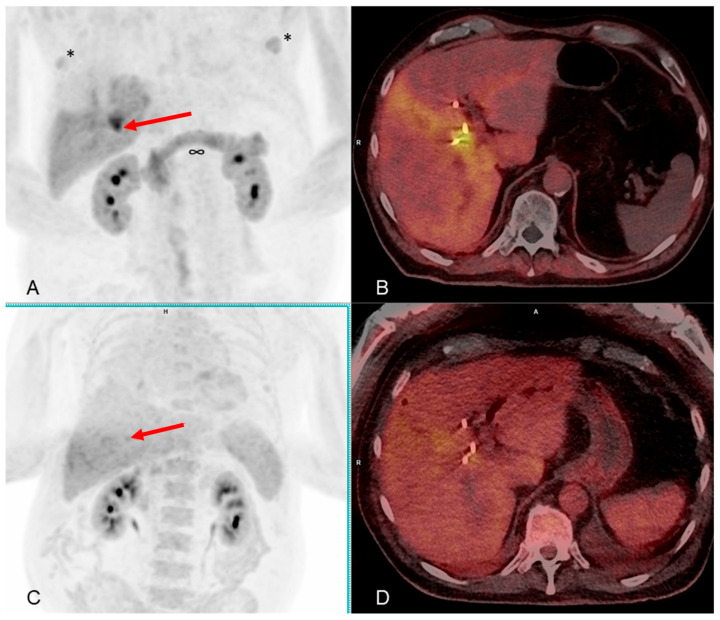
(A,B) MIP and hybrid PET/CT scan slice from December 2023 using [^68^Ga]Ga-FAP-2286: the red arrow marks an intense focal tracer uptake, which has been interpreted as the primary tumor lesion atop the confluence of the right and left hepatic bile ducts (Klatskin tumor). SUV_max_ 13.7; SUV_peak_ 10.7. (C,D) MIP and hybrid PET/CT scan slice from November 2023 using [^18^F]FDG: the red arrow marks a slight, inhomogeneous background noise enhancement at the confluence of the right and left hepatic bile ducts. However, the entire liver tissue shows this inhomogeneous uptake pattern. There is no clear focally-increased, neoplastic uptake delineated. Please take note of the liver parenchyma’s unevenly dispersed FAP expression, which has been interpreted as reactive uptake after acute cholangitis. It is important to take attention of the diffuse expression of FAP in the pancreas (∞), which has been attributed to a reactive inflammatory process following ERCP pancreatitis. Uptake in gynecomastic tissue is indicated by the *.
